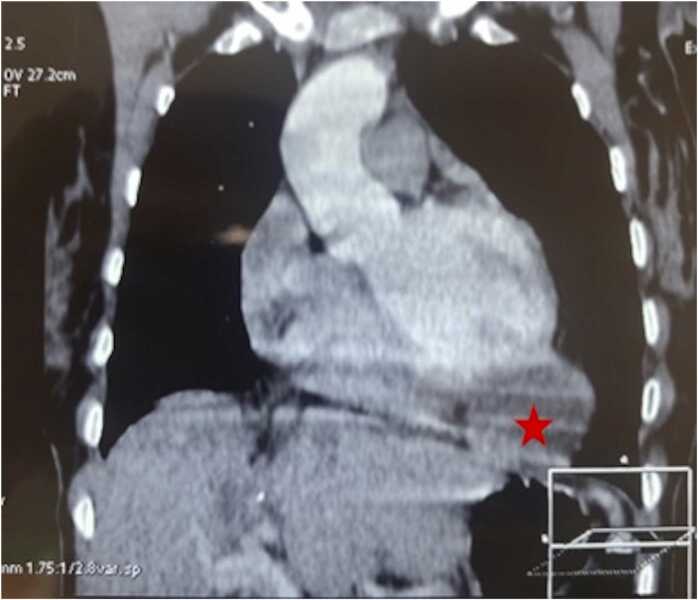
Thoracic computed tomography (coronal reconstruction) showing a well-defined cystic lesion (red star) located at the apical region of the left ventricle.
